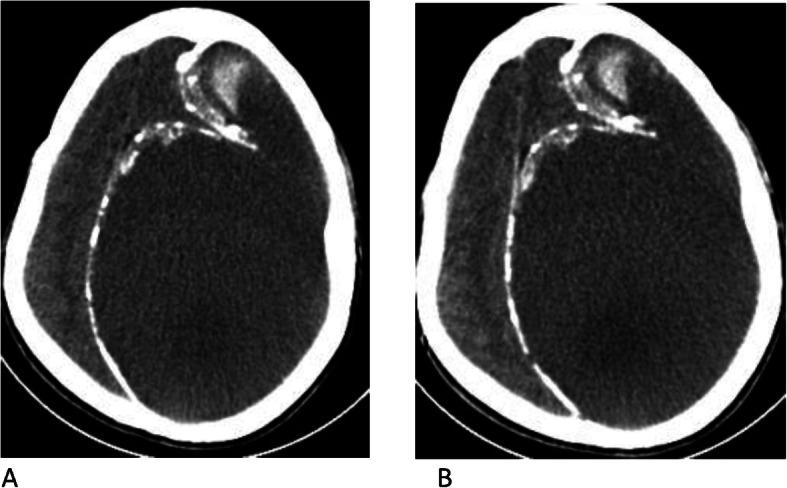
Pre- and postcontrast computed tomographic images. a Axial precontrast image showing left frontal, parietal, and temporal convexity calcified extra-axial subdural lesion with hypodensity and anterior focal areas of hyperdensity. b Axial postcontrast image showing no enhancement of the lesion
